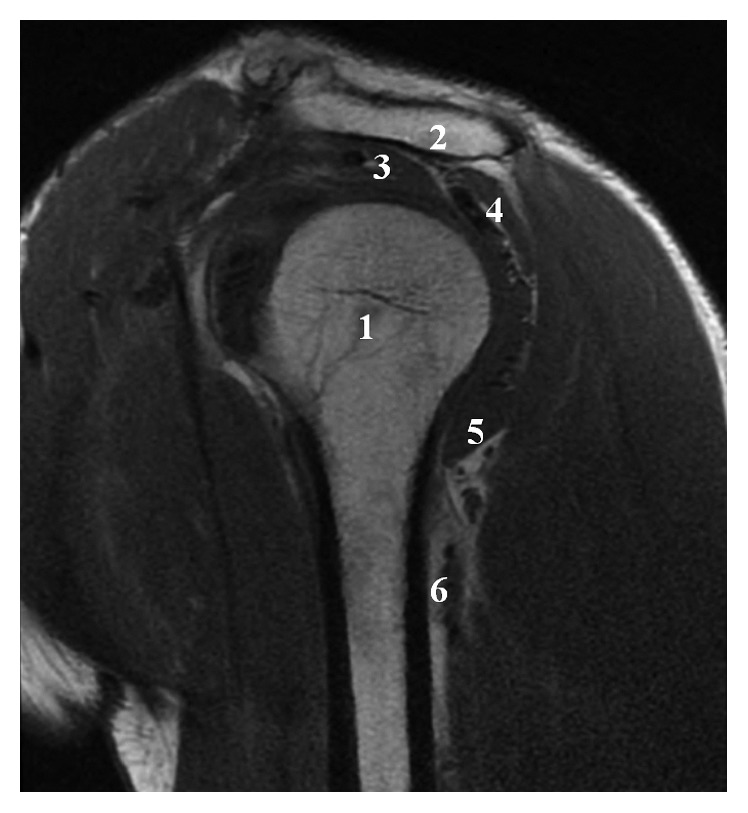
Sagittal T1 MRI: (1) humeral head, (2) acromion, (3) supraspinatus muscle, (4) infraspinatus muscle, (5) teres minor muscle, and (6) teres major rupture.
